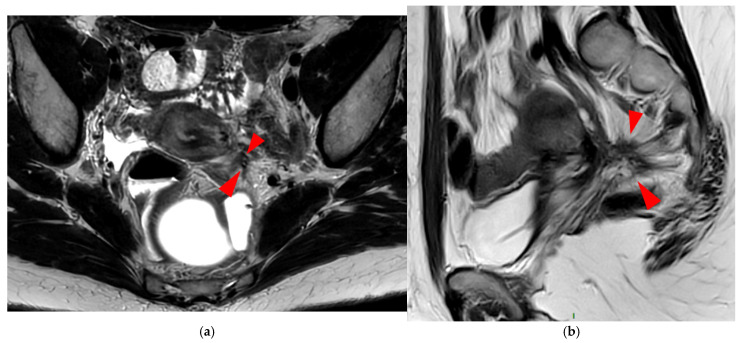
Pelvic MRI scan of a patient with a HTD type 6 left USL. (a,b) Axial (a) and sagittal (b) T2WI show a spiculated nodular hypointense lesion (*arrowheads*) extensively involving the left inferior hypogastric plexus contained within the sacro-recto-genital septum, as well as its afferent and efferent nerve fibers.
